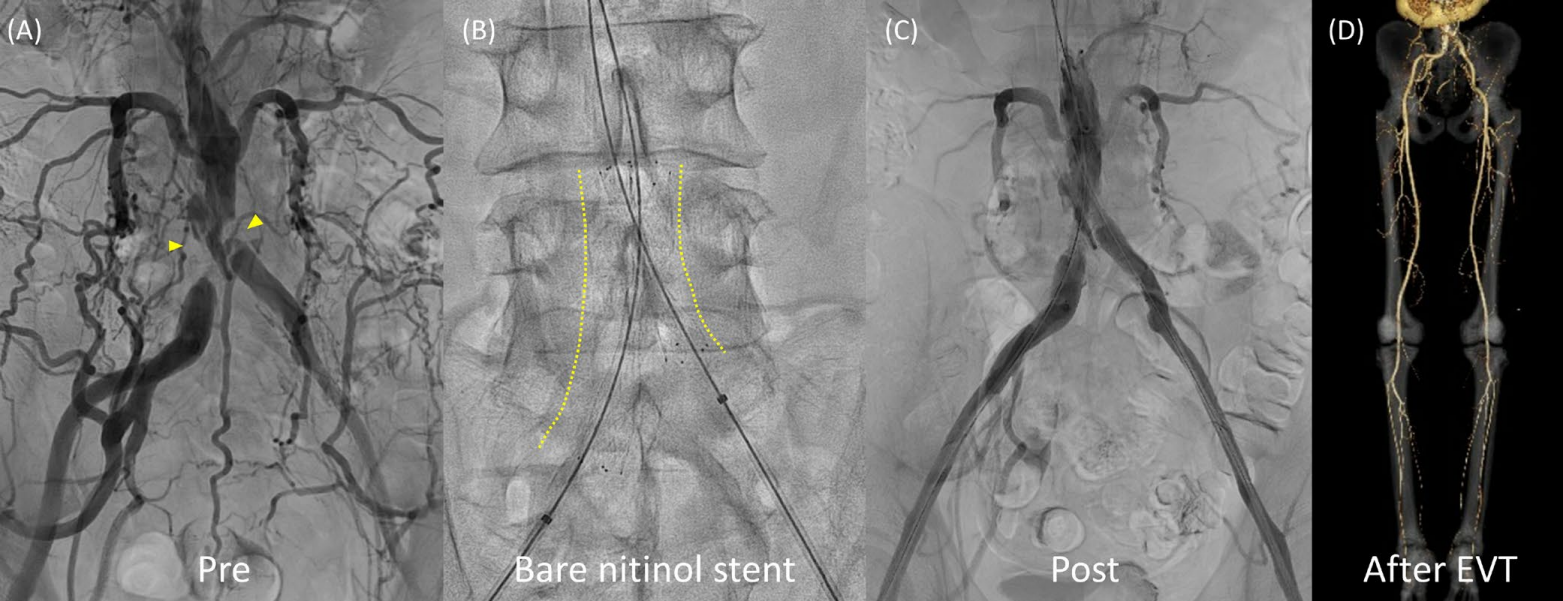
(A) Angiography showing occlusion of the terminal aorta and bilateral common iliac artery ostia (arrowheads). (B) Two bare nitinol stents deployed from the abdominal aortic terminus to the bilateral common iliac arteries in a hugging configuration. (C) Improved peripheral blood flow. (D) Urokinase administration resolving peripheral blood clots, with subsequent contrast‐enhanced computed tomography demonstrating improved blood flow to the left lower extremity.
